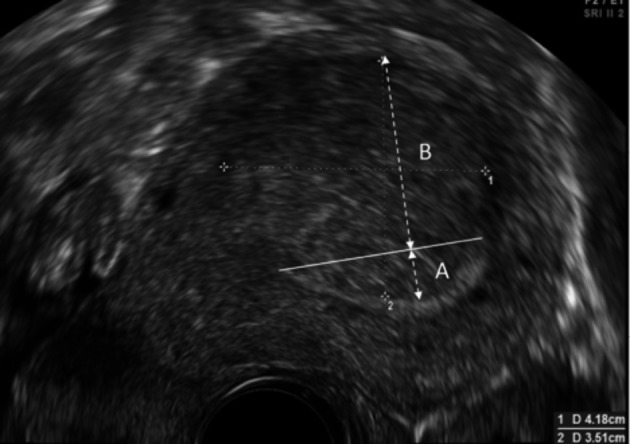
Ultrasound image of a submucous fibroid showing the subjective endometrial-myometrial junction (solid line), distance from the endometrial cavity (A) and extra-cavity size (B).
